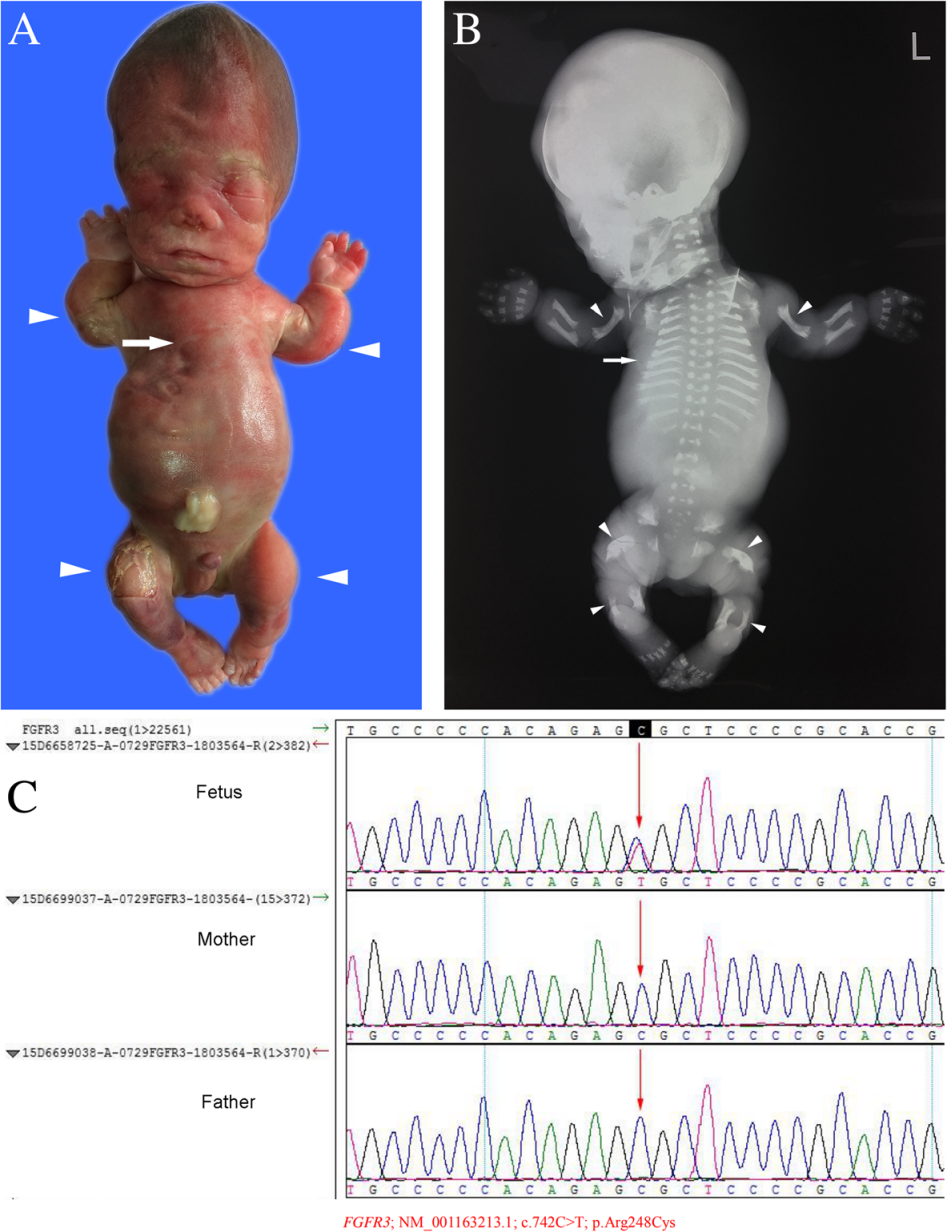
Case 26: The fetus with fatal achondroplasia and fibroblast growth factor receptor 3 (FGFR3) gene mutations. a Gross anatomy of the aborted fetus, showing the markedly short arms and legs (arrowheads), with a narrow chest (arrow). b X-ray images of the aborted fetus, showing the “telephone receiver” appearance of the femurs and humeri, the malformation of the metaphyseal (crateriform) (arrowheads), and the narrow thorax (arrow). c The fetus carried the c.742C > T (p.Arg248Cys) mutation in the *FGFR3* gene. The Sanger verification revealed that this mutation was new, and was not carried by the parents
